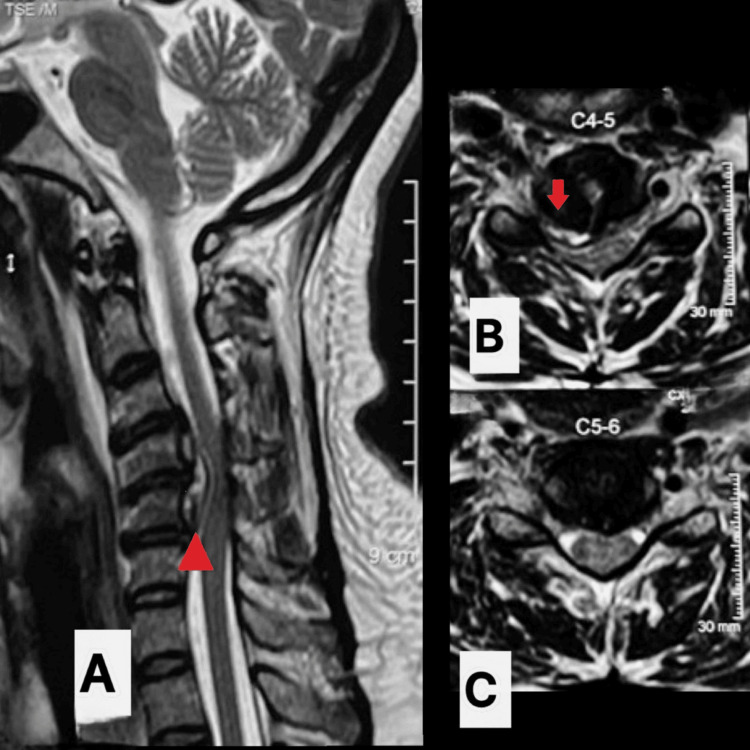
Preoperative T2w cervical MRI showing C4-5 disc signal changes in sagittal section (A) and axial section (B-C) with collection in the anterior epidural space descending behind the C5 vertebral body (marked with a red arrow). MRI: magnetic resonance imaging, T2w: T2-weighted, C4-5: cervical 4-5, C5: cervical 5
